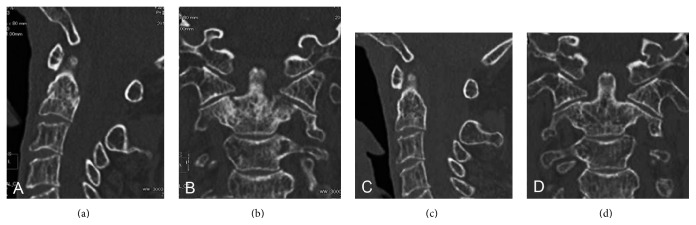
Sagittal (a, c) and coronal (b, d) reconstruction images of computed tomography (CT). (a, b) Anderson type III odontoid fracture was confirmed at the time of initial diagnosis. (c, d) Follow-up CT obtained after 6-month conservative treatment clearly revealed complete bone union.
